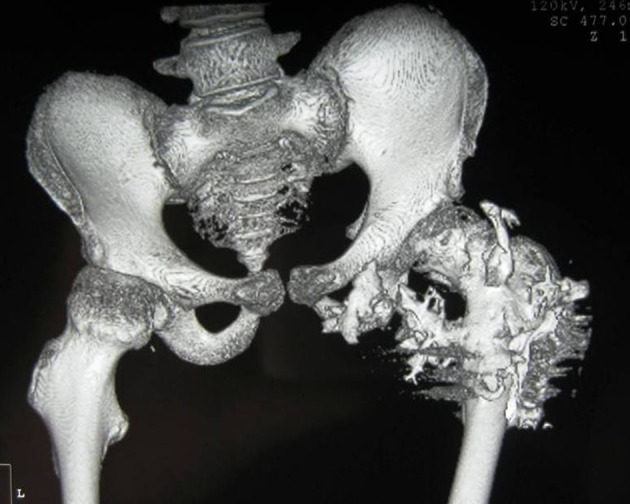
3D reconstruction CT scan of the pelvis in a 15-year-old female patient showed massive heterotopic ossification around the pelvis and the upper shaft of the left femur and the ischium pubis with eventual development of extra-articular ankyloses (firm bridging from the femur to the pelvis) associated with massive ossification of the soft tissue (i.e. heterotopic ossification formed in the soft tissues above and below the greater trochanter of the femur).
